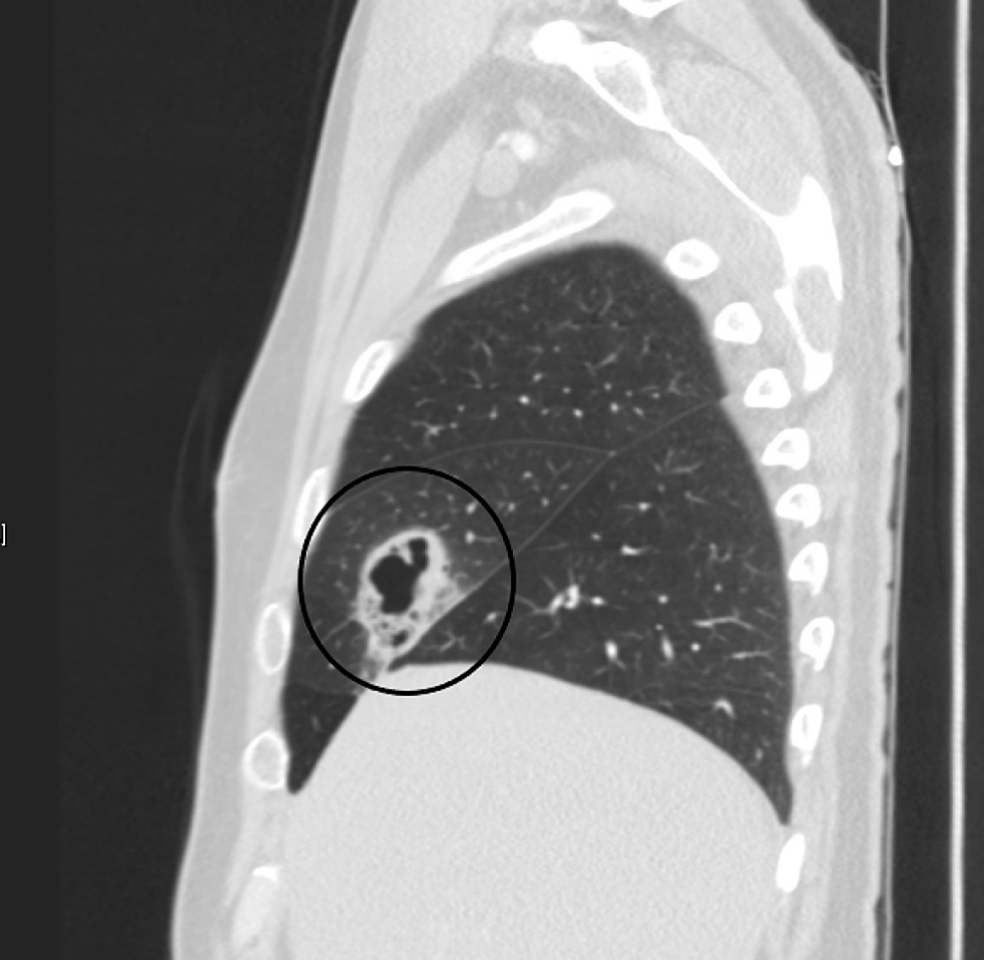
CT of the chest showing a 4.0 x 4.6 cm cavitating lesion in the right middle lobe with associated, surrounding ground-glass opacities in the coronal plane.
